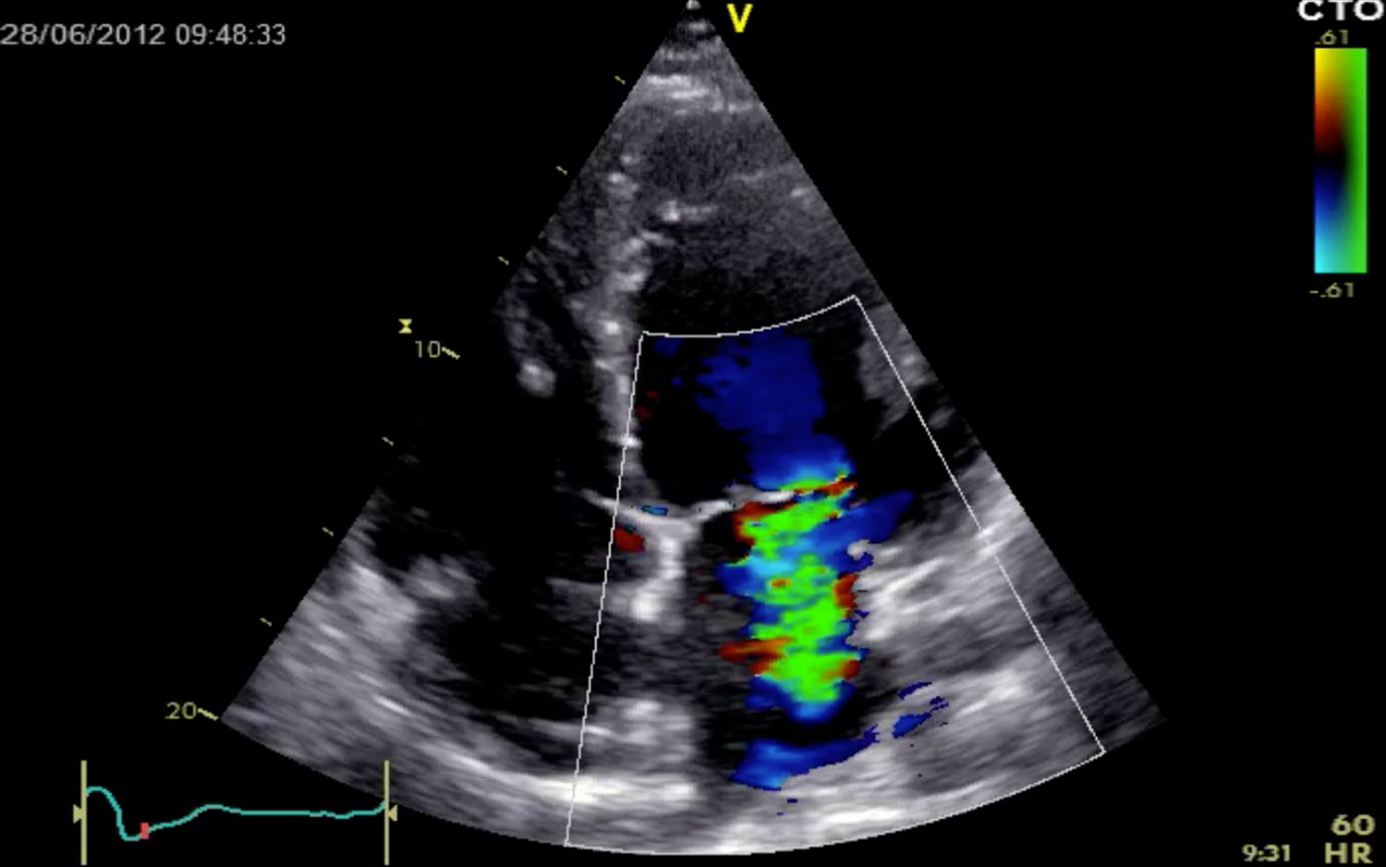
Mitral valve regurgitation, with doppler, via 4C view
